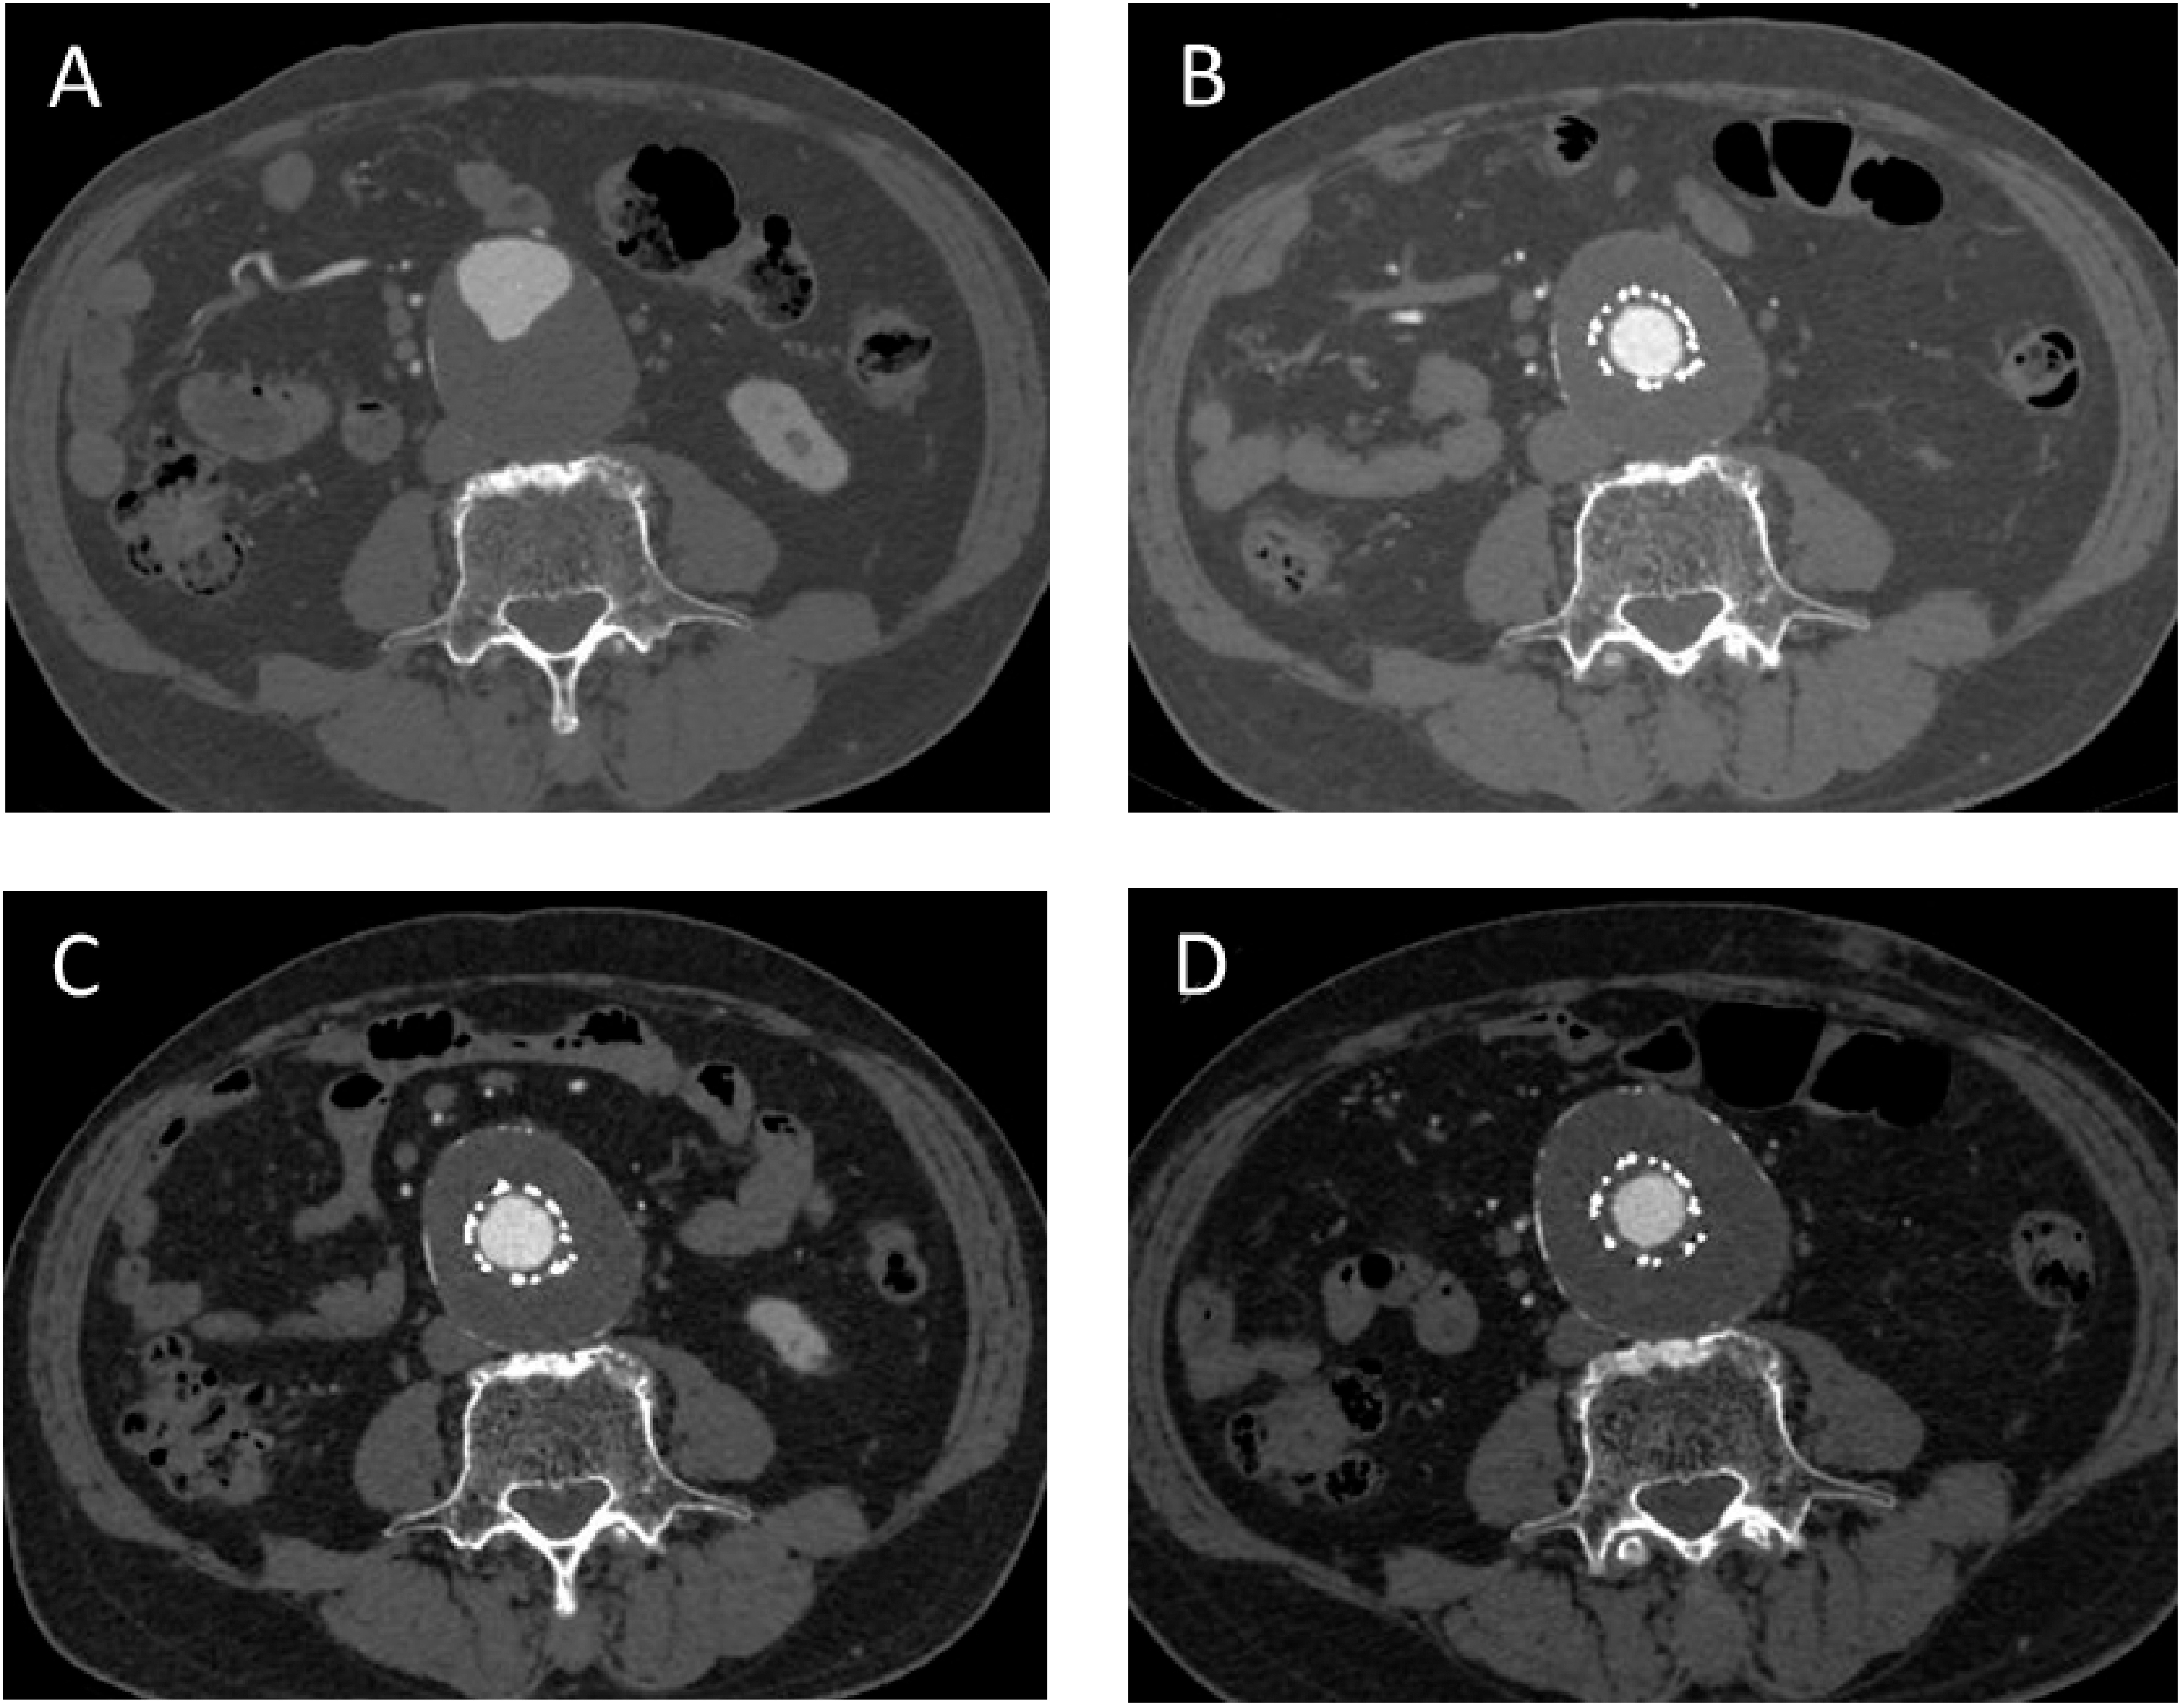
Fig. 1 Contrast-enhanced computed tomographic scan showing (A) a maximal aneurysm diameter of 65 mm before endovascular aortic repair (EVAR), (B) a maximal diameter of 65 mm with no signs of endoleak at 6 months after EVAR, (C) an unchanged maximal diameter of 66 mm with no signs of endoleak at 12 months after EVAR, and (D) an increased maximal diameter of 73 mm with no signs of endoleak at 24 months after EVAR.
